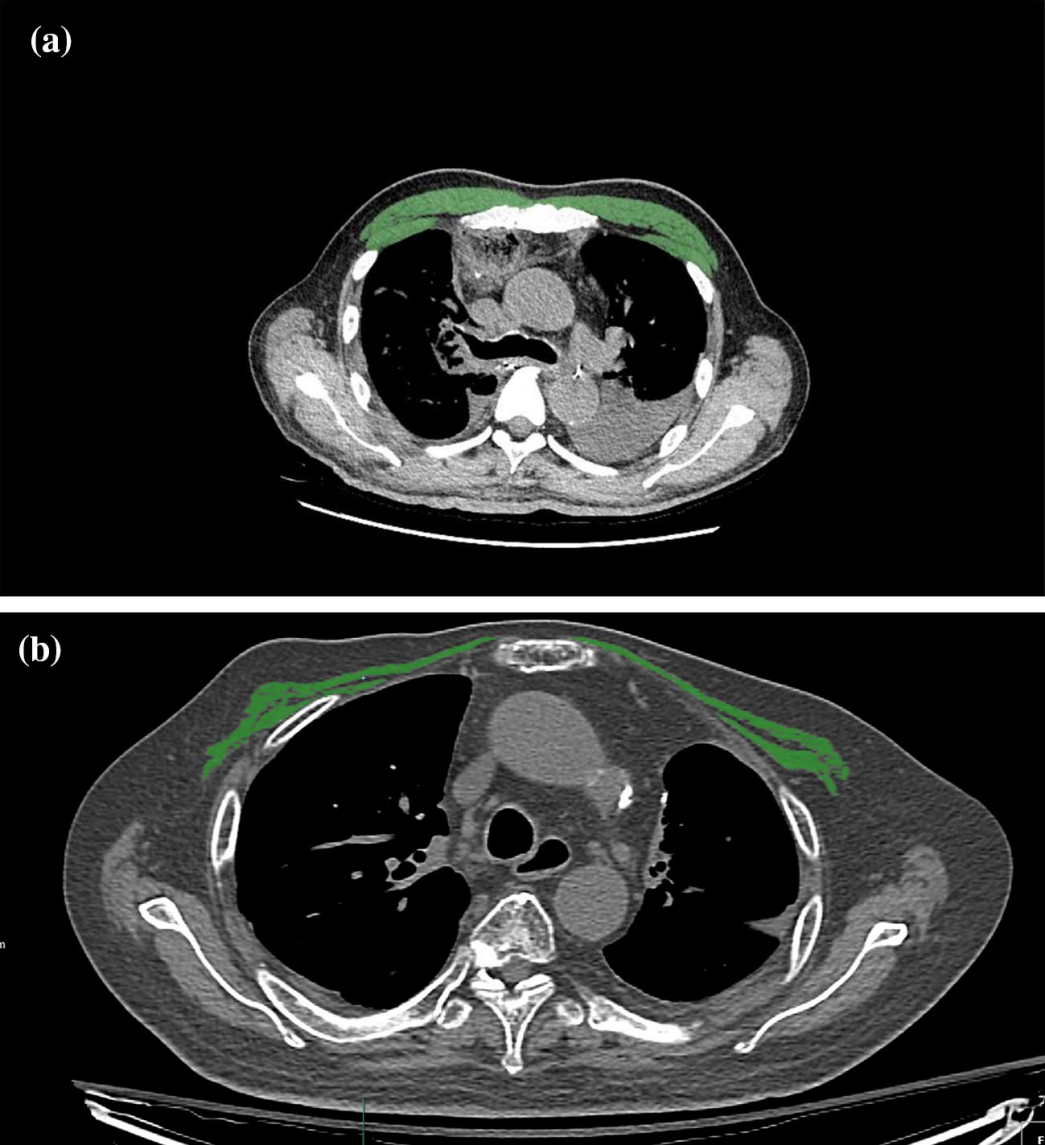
Sample axial computed tomography (CT) images of the fourth thoracic vertebral region. (a) A patient with PM_CSA_ values above the median. (b) A patient with PM_CSA_ values below the median. Images are used to determine the muscle area in elderly ICU patients. The pectoralis muscles are shown in green.
